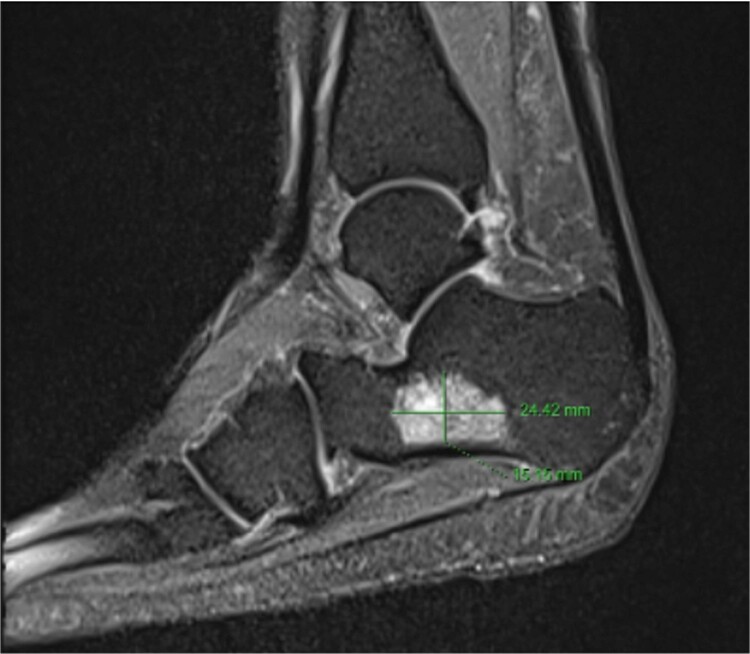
A 15 × 24-mm tumor in the right calcaneus was prominently visible in the MRI images, with irregular margins, strong enhancement, and no invasion beyond the bone.
